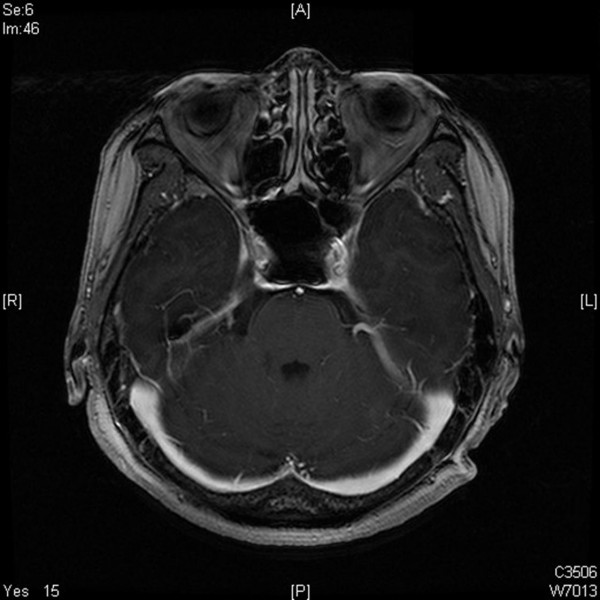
Following six cycles of chemotherapy, axial MRI revealed no enhancing lesion in the sphenoid and cavernous sinuses.
